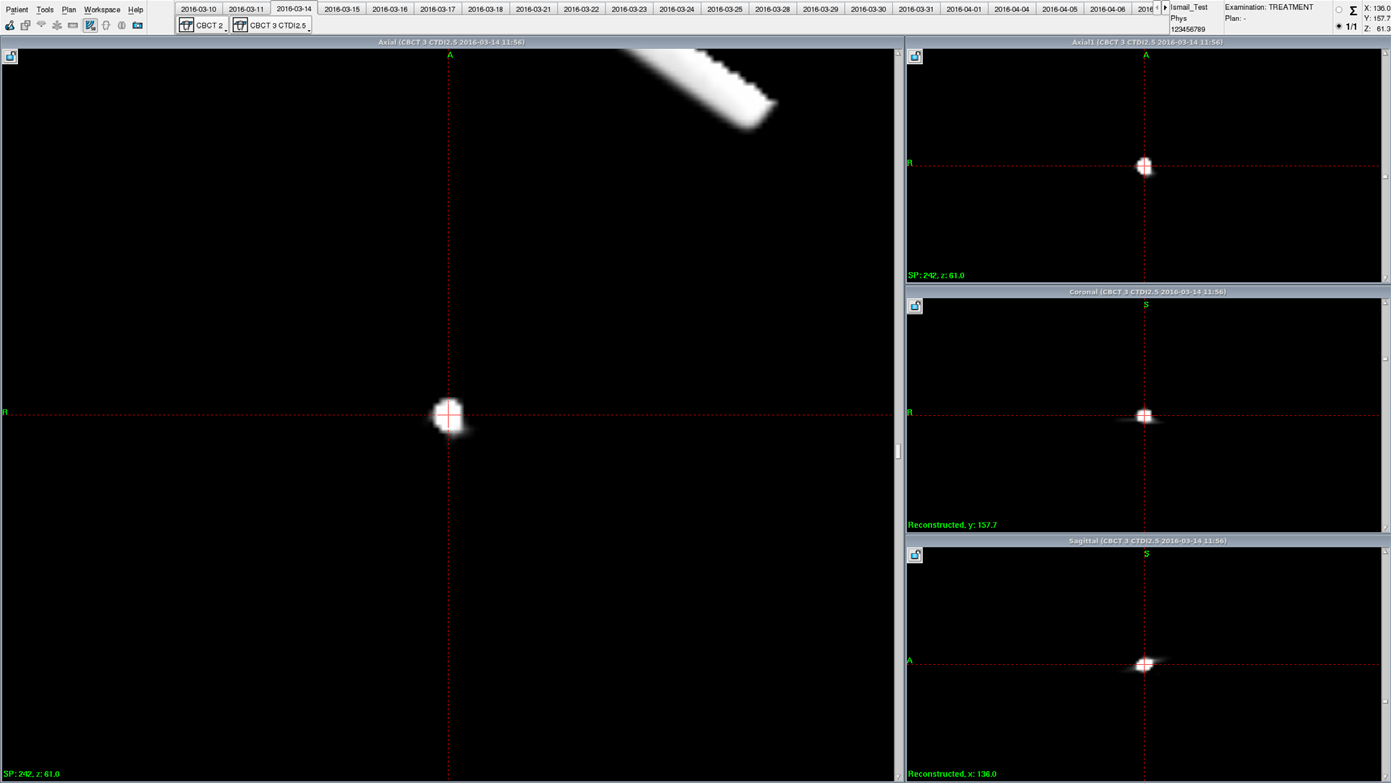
A snapshot of the Leksell GammaPlan treatment planning software showing a zoomed‐in and maximally contrasted CBCT image of one of the markers (marker (a) is shown here). The coordinates reported for each marker were the average of three readings of the geometrical center of markers as determined visually by a single observer.
